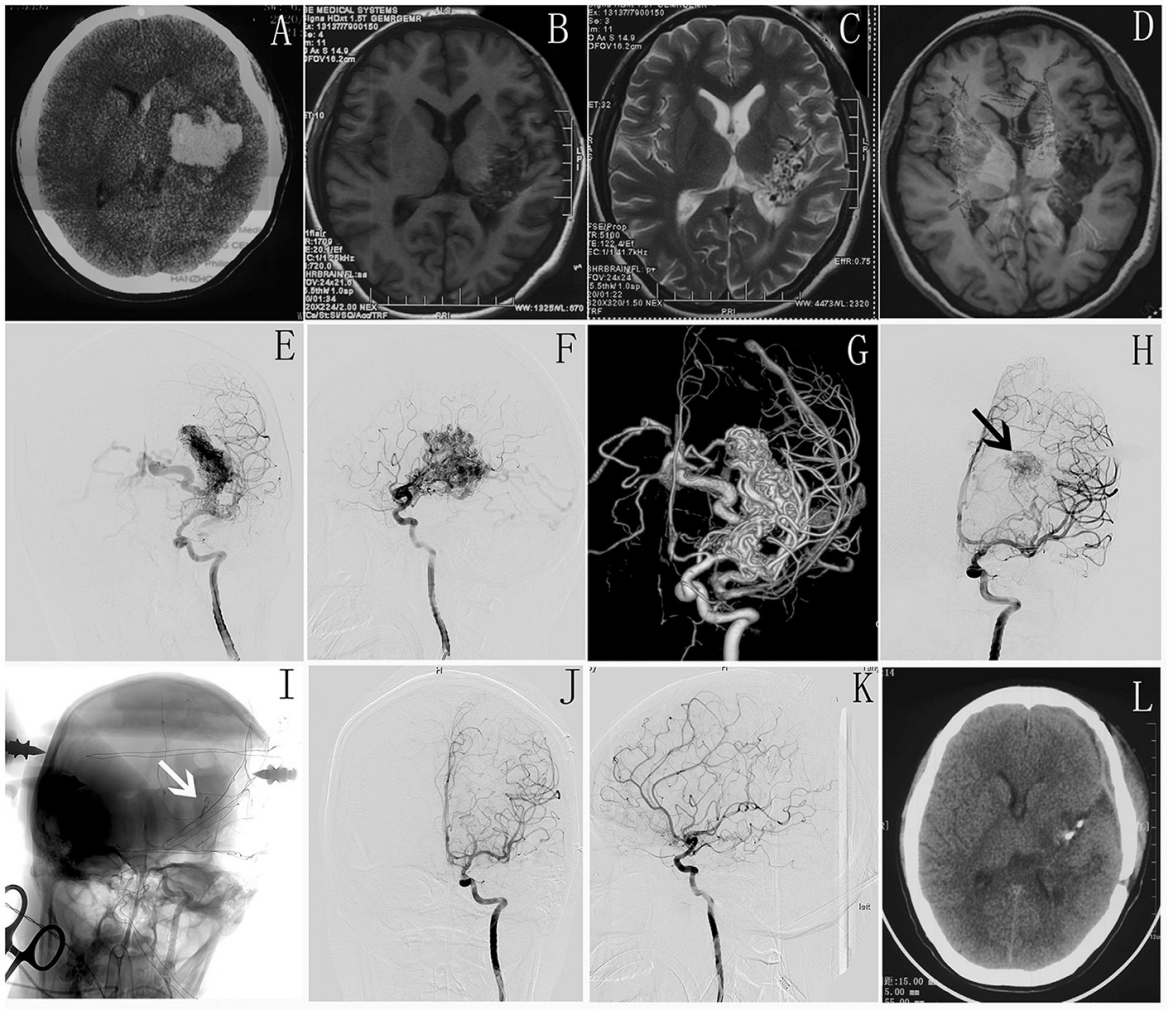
(A) Preoperative CT shows intracranial hematoma in left basal ganglia. (B–D) MR scan shows malformed vascular mass in left basal ganglia and some damage in corticospinal tract. (E–G) Intraoperative DSA shows AVM located in basal ganglia. (H,I) Intraoperative DSA shows the residual AVM nidus (white arrow) and a clipper used as markers to accurately locate the residual AVM lesion(black arrow). (J,K) The immediate postoperative DSA shows complete occlusion of AVM. (L) postoperative CT scan shows no more damage in the normal brain tissue.
